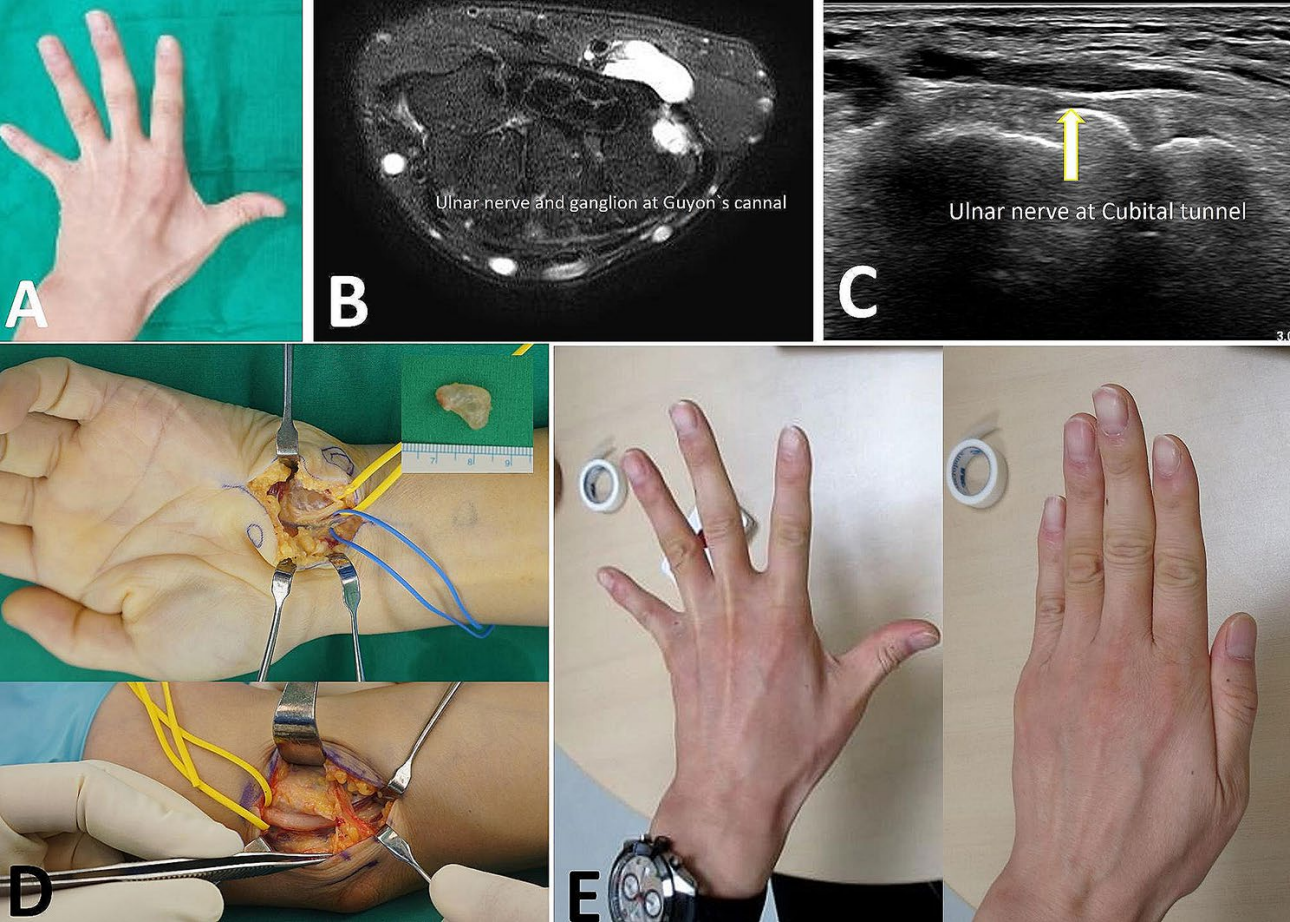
A forty-seven years old male came for left 2nd finger weakness after golf. Double crush syndrome was confirmed by EMG/NCS with Cubital tunnel syndrome (on left elbow) and Guyon’s canal syndrome (on left wrist). (A) On physical examination, 1st web space atrophy was observed., (B) The preoperative T2 magnetic resonance images of left wrist show a 1.5 cm sized ganglion mass in Guyon’s canal of hypothenar muscle layer. (C) The preoperative ultrasonography of left wrist view shows ulnar nerve swelling and a cystic lesion in Guyon’s canal (white arrow). (D) Intraoperative exploration, the ulnar nerve was compressed by huge ganglion at left wrist and below left elbow medial epicondyle, the ulnar nerve was compressed with swelling. (E) On physical examination of last follow up, 1st web space atrophy was improved. (Mcgowan stage 0)
